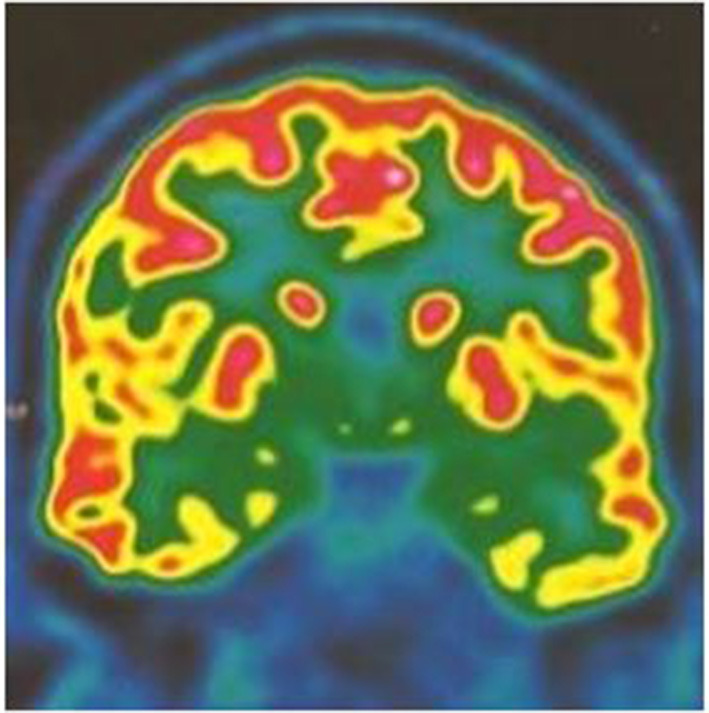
18 FDG PET scan coronal cut showing bilateral mesial temporal hypometabolism more pronounced on the left temporal lobe.
